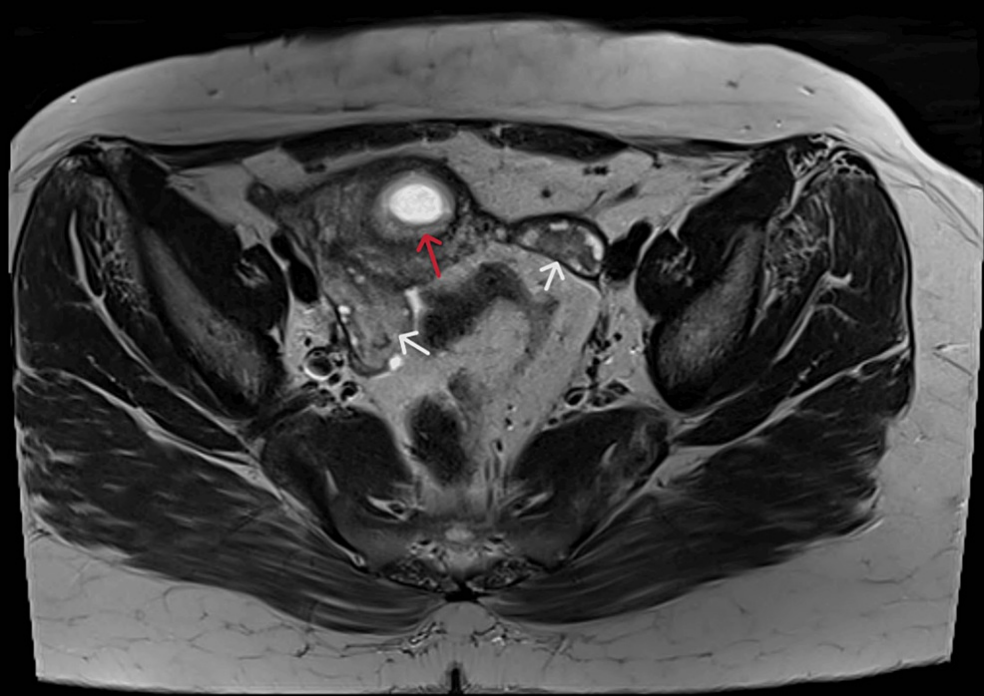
T2-weighted axial magnetic resonance imaging with the red arrow representing the gestational sac.
